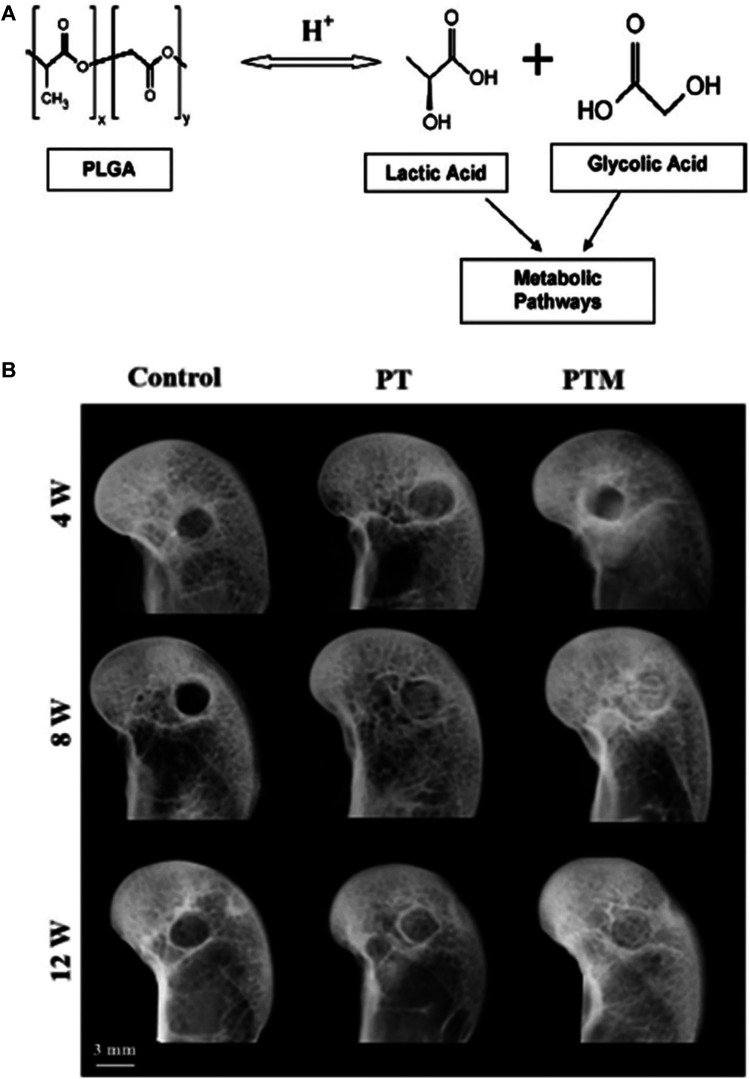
PLGA for osteonecrosis therapy. (A) Hydrolysis of PLGA (Danhier et al., 2012). (B) Representative radiographs showed that new bone formation within bone tunnel at 4, 8, and 12 weeks after surgery (Lai et al., 2019). Reproduced with permission (Danhier et al., 2012). Copyright 2012, Elsevier Ltd. Reproduced with permission (Lai et al., 2019). Copyright 2019, Elsevier Ltd.
